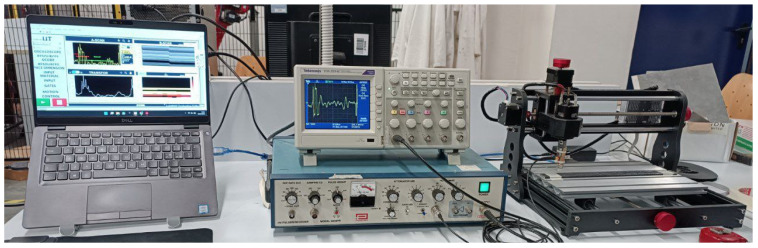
Ultrasonic inspection equipment.
